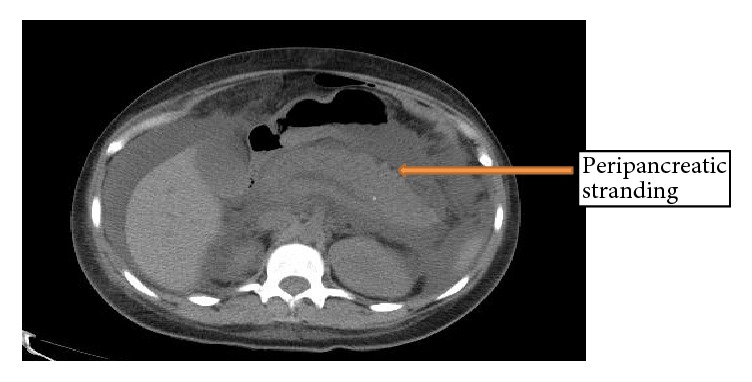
CT scan of the abdomen showing acute pancreatitis (arrow).
